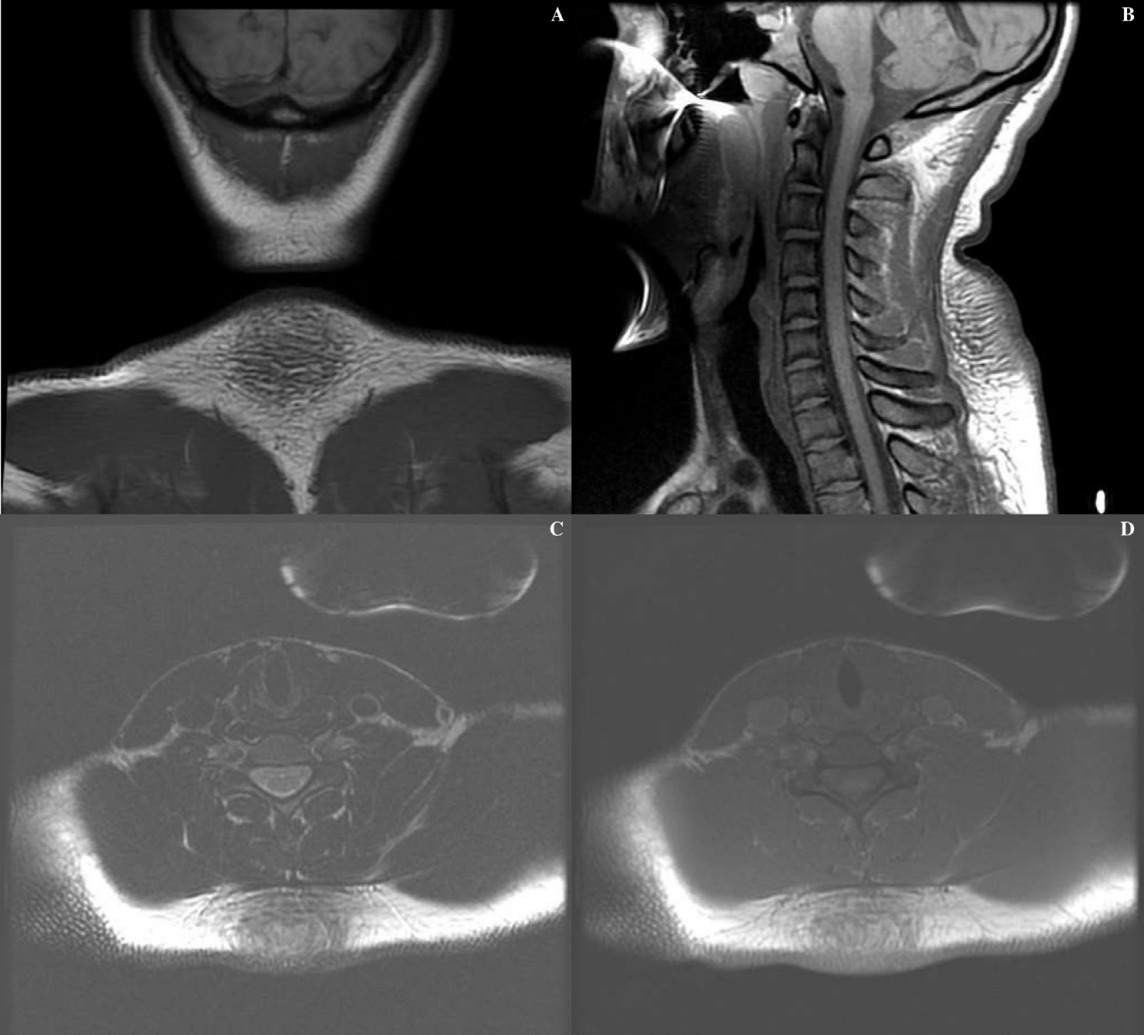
T*_1_ weighted non-contrasted MRI of the cervical
